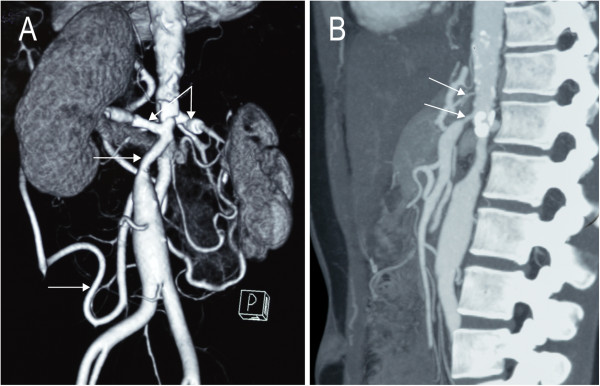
Preoperative imaging showing multiple arterial stenoses. A, Posteroanterior view of three-dimensional computed tomography angiography showing coarctation of the abdominal aorta (middle arrow), bilateral renal artery stents (two upper arrows) and arc of Riolan (lower arrow). B, Superior mesenteric artery stenosis (lower arrow) and celiac artery stenosis (upper arrow).
